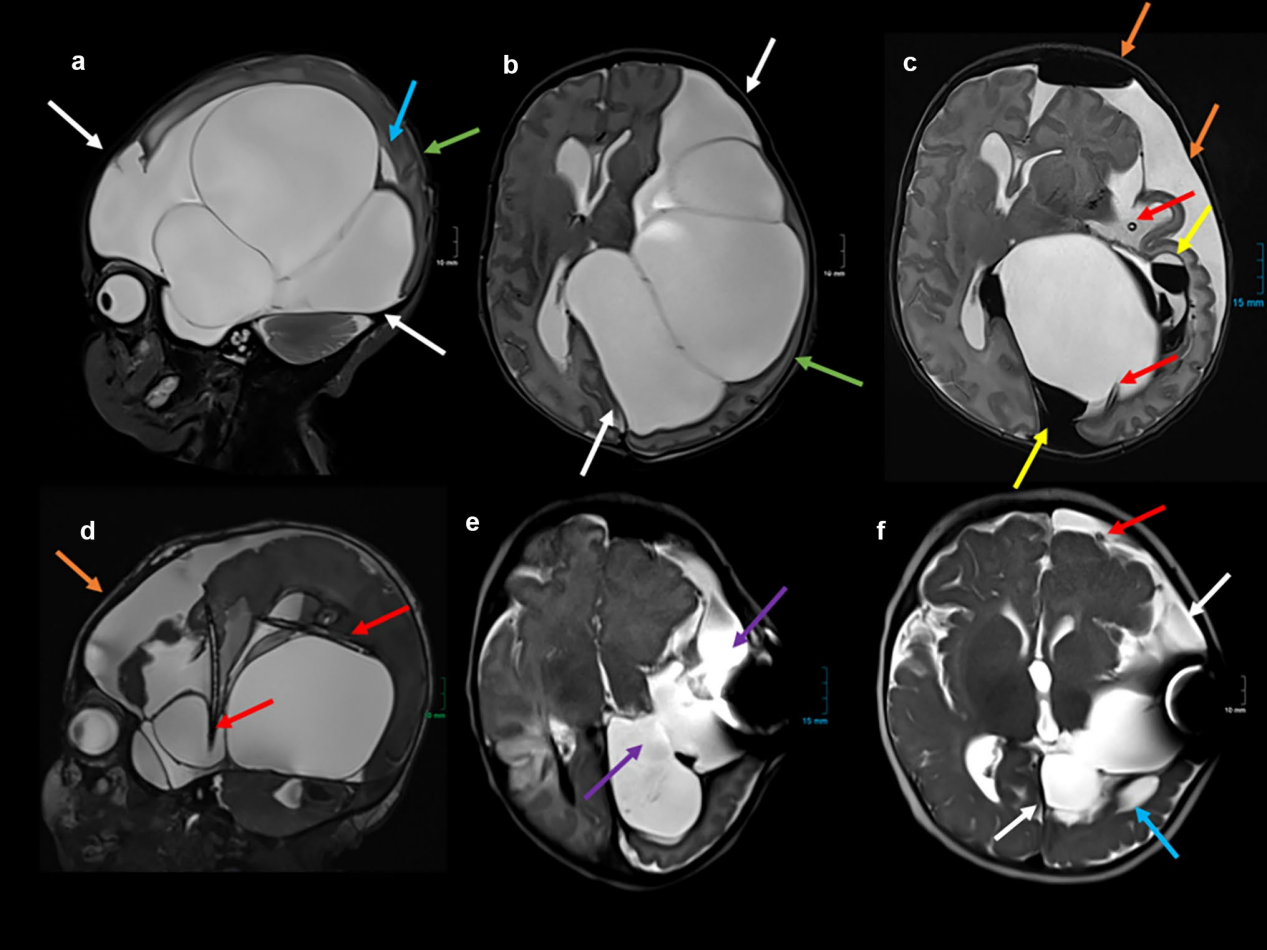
a, b Pre-surgical MRI (T2 TSE in sagittal (a) and axial (b) orientations): several large cysts occupying most of the left hemispheric space (white arrows). Dislocation of the left temporal lobe (a, b, green arrow) and left temporal horn (a, blue arrow). c, d MRI after surgery (c: T2 TSE in axial orientation, d: T2 CISS in sagittal multiplanar reconstruction): two ventricular catheters in several compartments of the cystic formation (red arrows) with some amount of hemorrhage (yellow arrows), left frontal hygroma (orange arrows) with pneumatocephalon. e MRI (T2 HASTE in axial orientation) after microsurgical marsupialization of the cyst walls with consecutive communication of the compartments with each other (purple arrows). f Follow-up MRI (T2 HASTE in axial orientation) at the age of 7 months: some residuals of the cysts (white arrows), left temporal horn still dislocated (blue arrow), residual left frontal hygroma with subduro-peritoneal shunt (red arrow)
